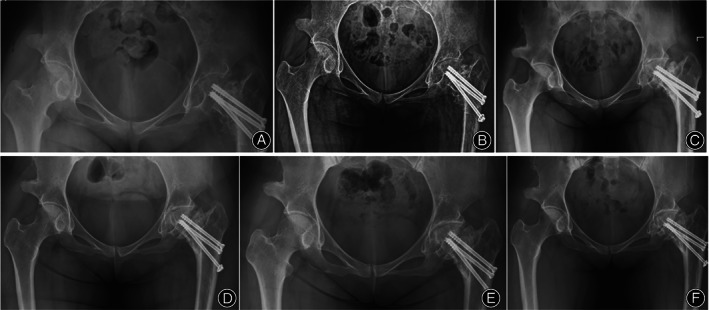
patient's orthopedic pelvic radiographs 1 month (A), 3 months (B), 1 year (C), 3 years (D), 5 years (E), 7 years (F) after surgery.
